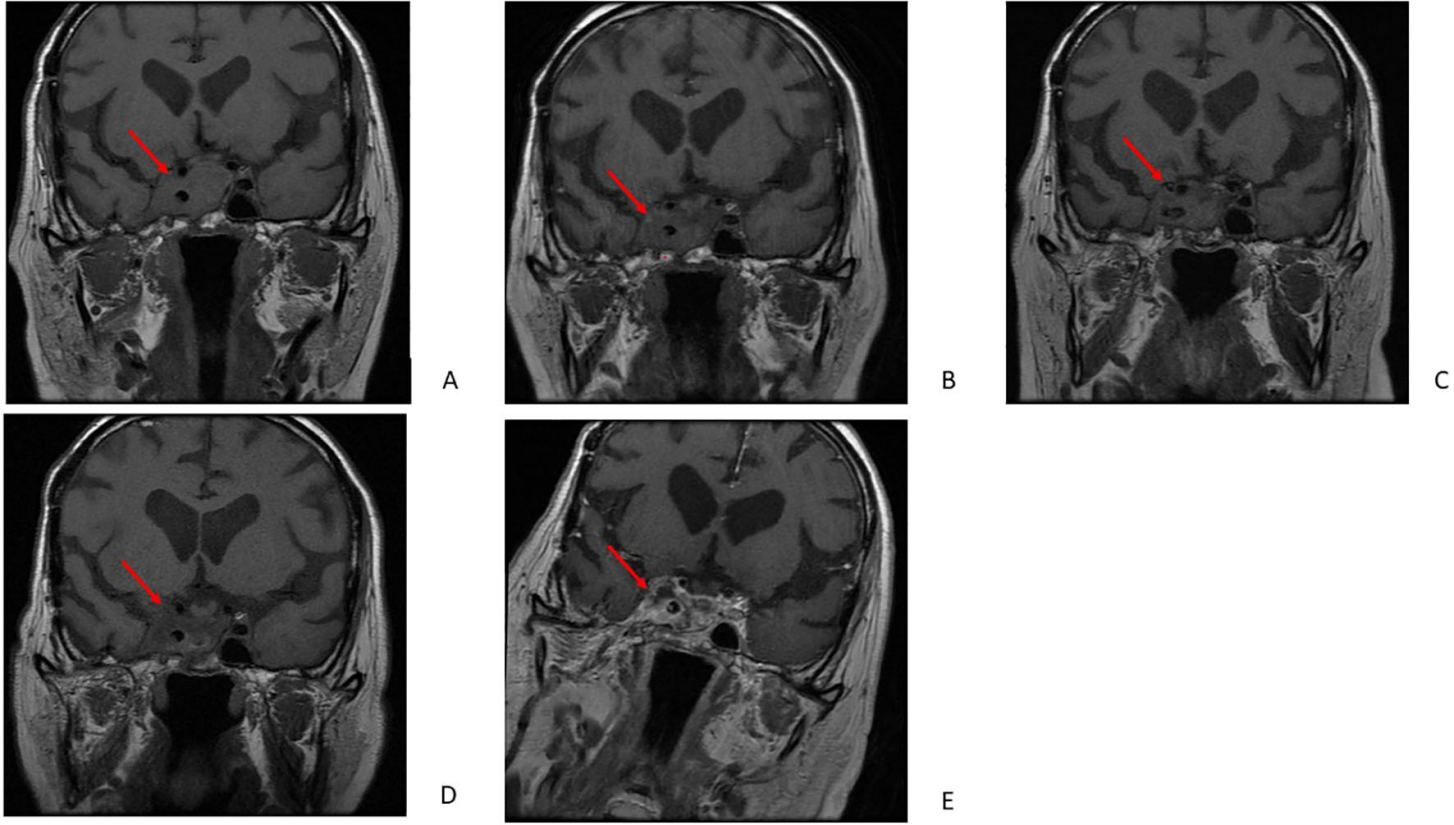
MRI examinations. The images show the patient’s coronal MRI of the pituitary tumor in July 2018 (A), December 2019 (B), February 2021 (C), March 2022 (D), and August 2022 (E).
